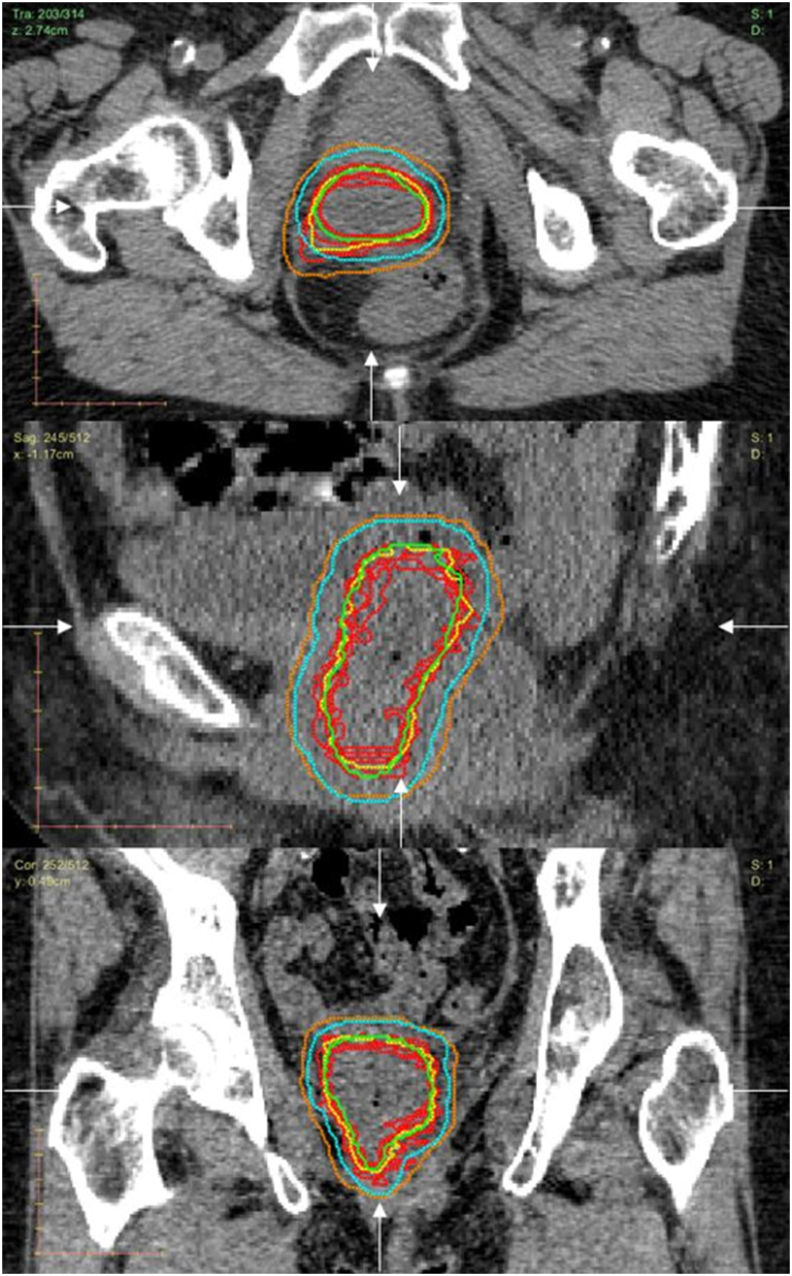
A selection of the outlines for patient 14; computed tomography–magnetic resonance imaging (CT-MRI) gross tumour volume (GTV) outlines from each observer are shown in red; CT-MRI GTV consensus outline is shown in yellow; MRI consensus outline is shown in green; CT-MRI planning target volume (PTV) is shown in orange; finally the MRI PTV is shown in turquoise. The locations of the viewing planes are indicated by the white arrows.
